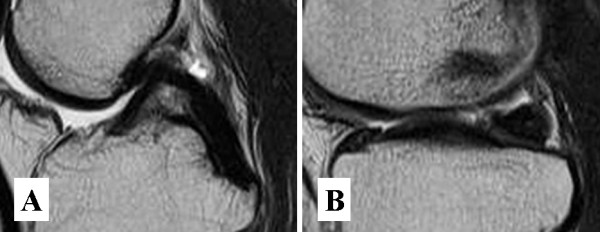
Sagittal image of T2 weighted MRI examined 9 months after the operation. The meniscal cyst has completely disappeared (A). Note that grade 2 signal intensity inside the meniscus decreased compared with abnormal signal intensity shown in Fig 1-A (B).
